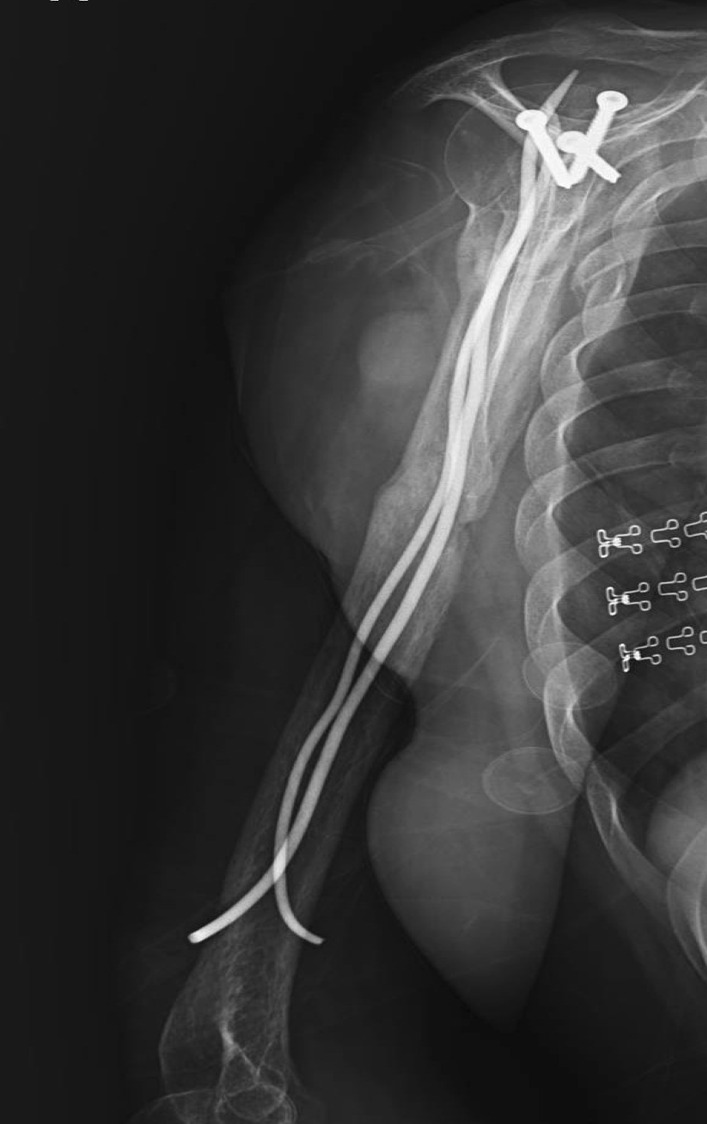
Lateral X-ray showing partial union in second postoperative month
